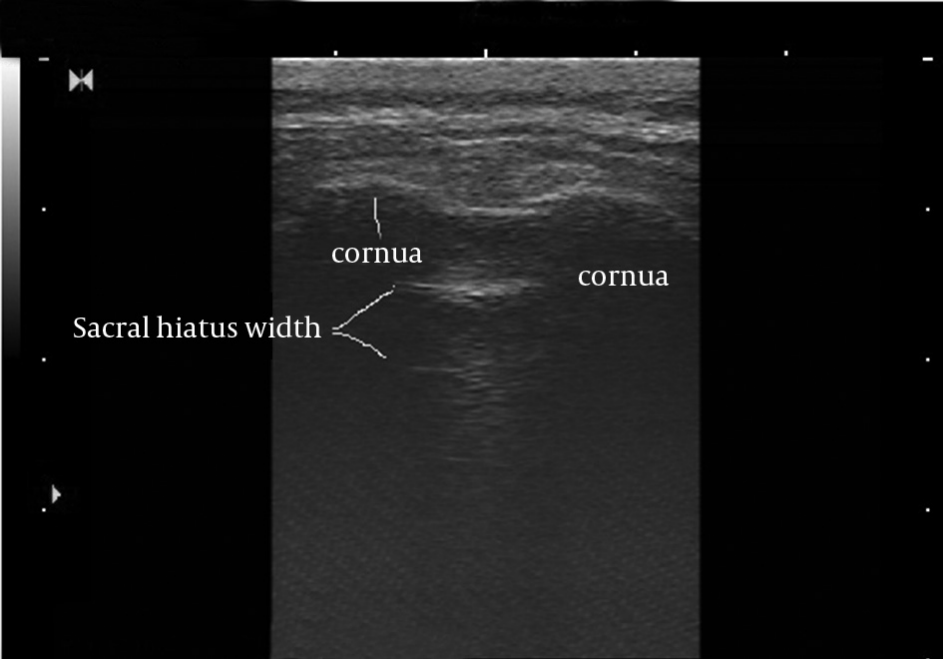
Transverse view of the sacral hiatus by ultrasound; the bilateral cornua are seen as reverse U-shaped structures.
